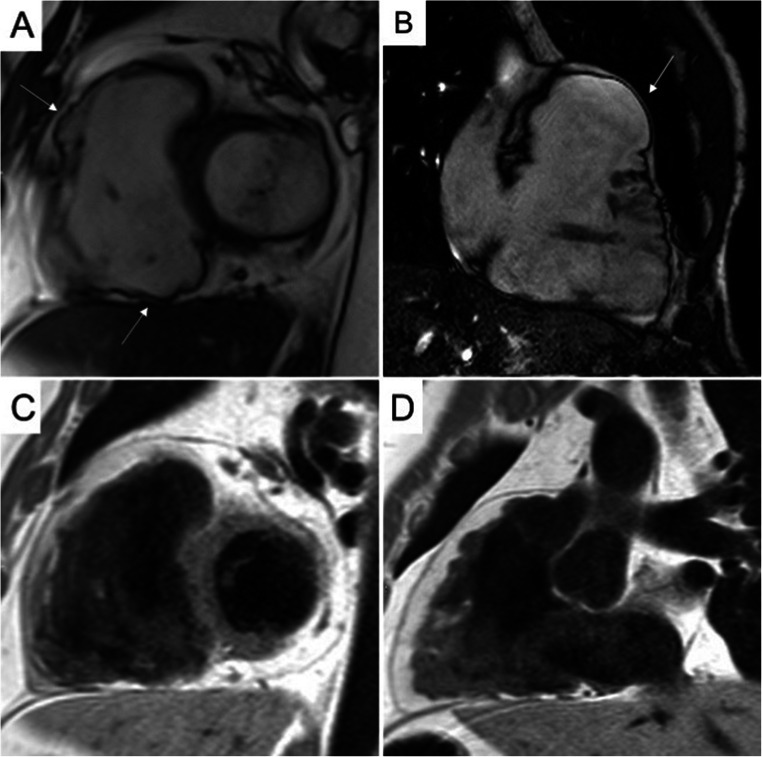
CMR images showing some peculiarities of a right dominant variant of ACM. A, B Cine image in short-axis view (systolic frame) showing multiple sacculations of the RV walls (white arrows). C, D T1-weighted images demonstrating massive fatty infiltration of the RV, with fat appearing as white tissue inside the RV wall. ACM, arrhythmogenic cardiomyopathy; RV, right ventricle
